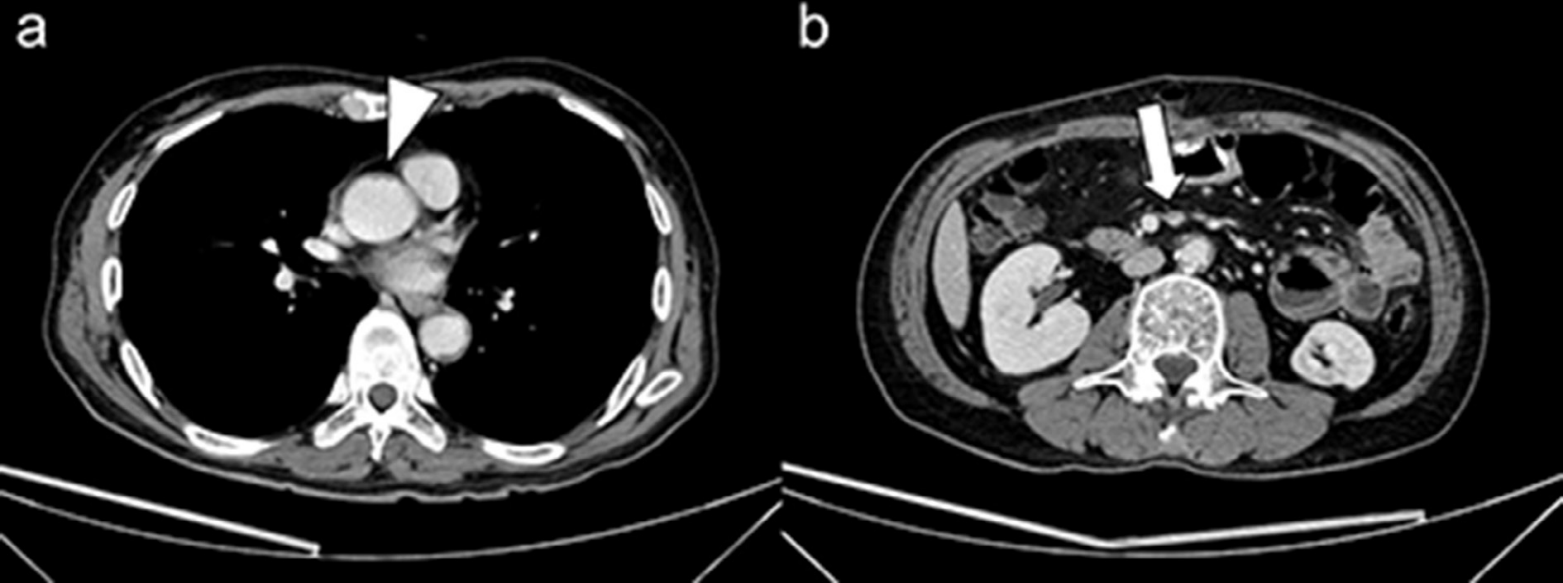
Contrast-enhanced CT shows resolution of the floating aortic thrombus (a) and reduction in the size of the SMA thrombus (b). Arrowhead indicates resolution of the aortic floating thrombus, and the arrow indicates reduction in the size of the SMA thrombus.
